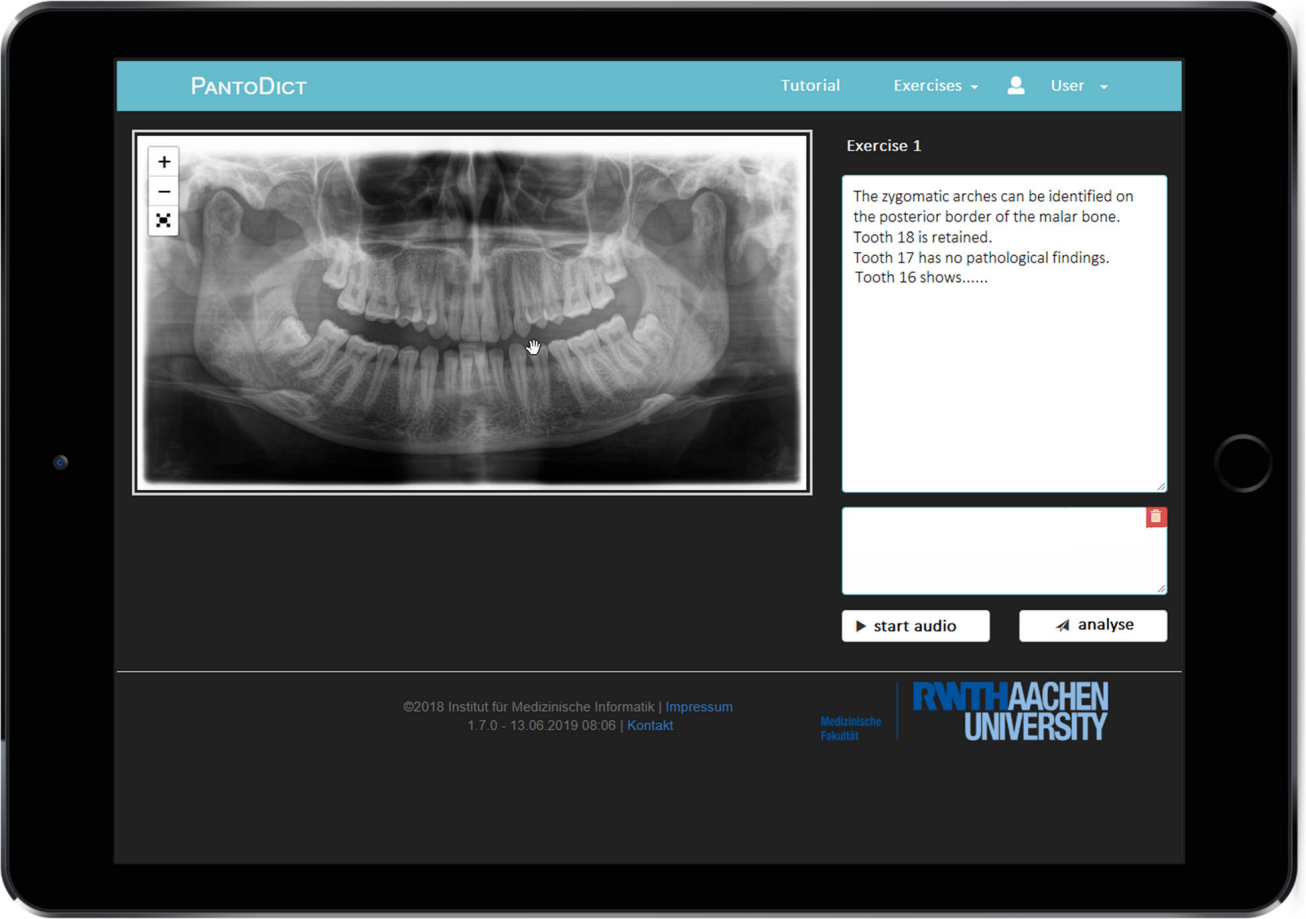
Screen view showing a reporting exercise in process. The radiograph can be zoomed in and out to observe details. The right upper text field contains the entered report. The second text field shows the recognized text while using automatic speech recognition and can also be used to correct the text. Once the report is completed, the analysation can by started by pressing the button‘analyse’
